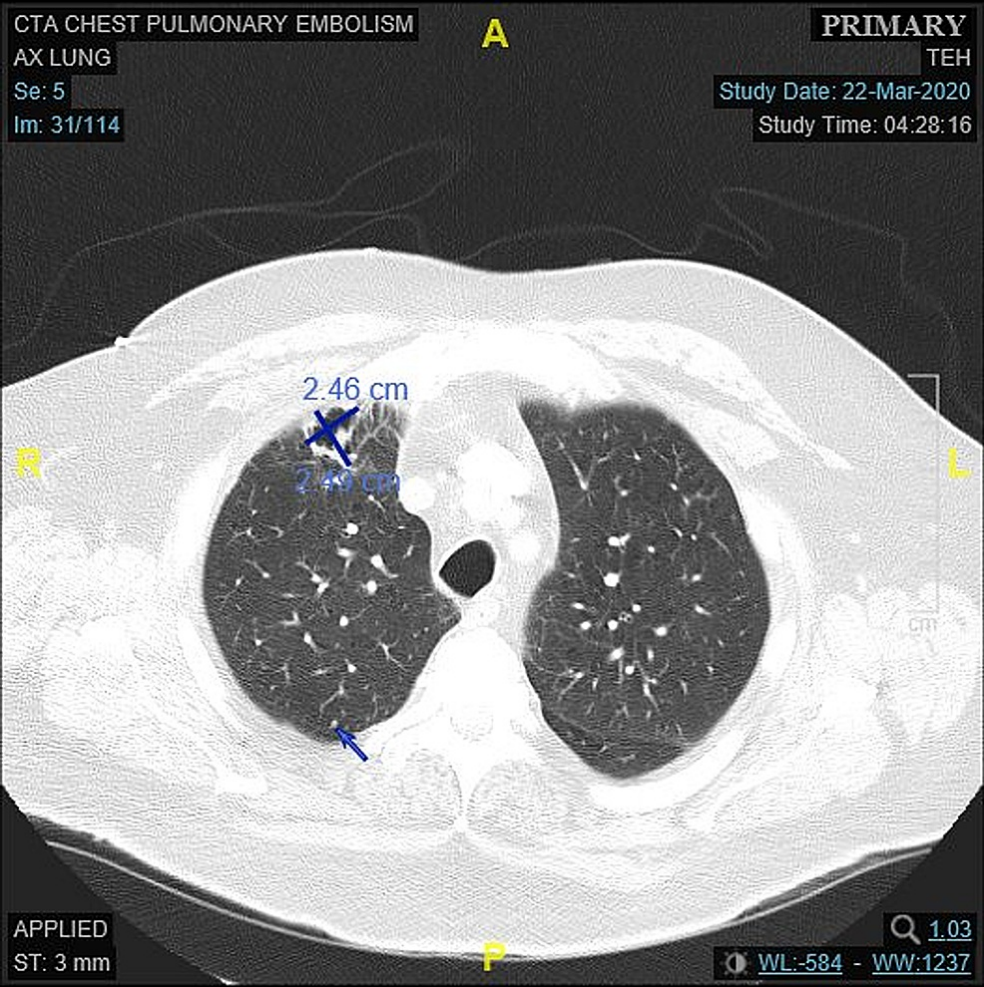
CT chest obtained approximately six weeks after the initiation of dabrafenib 150 mg BID/trametinib 2 mg daily combination therapy with near-complete resolution of the right pulmonary mass (size decreased from 5.02 cm x 5.49 cm to 2.46 cm x 2.49 cm) with central necrosis
